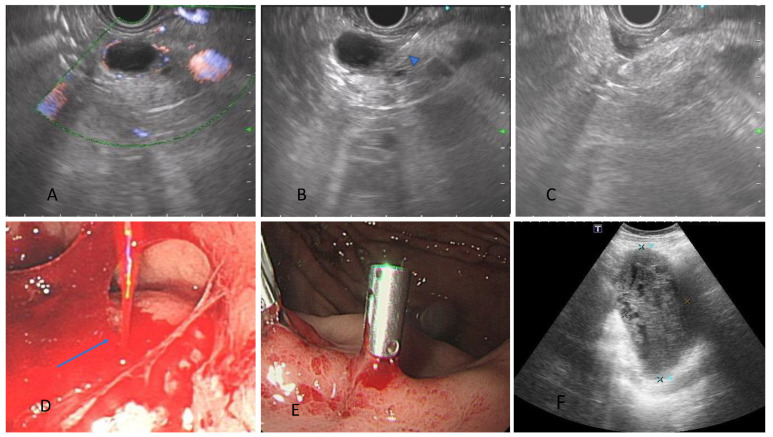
A case of severe bleeding after EUS-FNB in a patient with cystic pancreatic neuroendocrine tumor. (A) A cystic lesion 1.8 cm in pancreatic tail, with blood flow noted in the rim. (B) We punctured the thickened wall of the cystic lesion using 22 G Franseen-tip needle without penetrating intervening vessels (arrowhead). (C) Some fluid accumulation was visualized around the pancreas after tissue acquisition. (D) After shifting to the endoscopic view, a spurting vessel was noted (arrow). (E) Two hemoclips were applied right after EUS-FNB, and hemostasis was achieved. (F) Follow-up abdominal sonography 1 month later showed a huge intra-abdominal hematoma, 14 cm × 5 cm.
